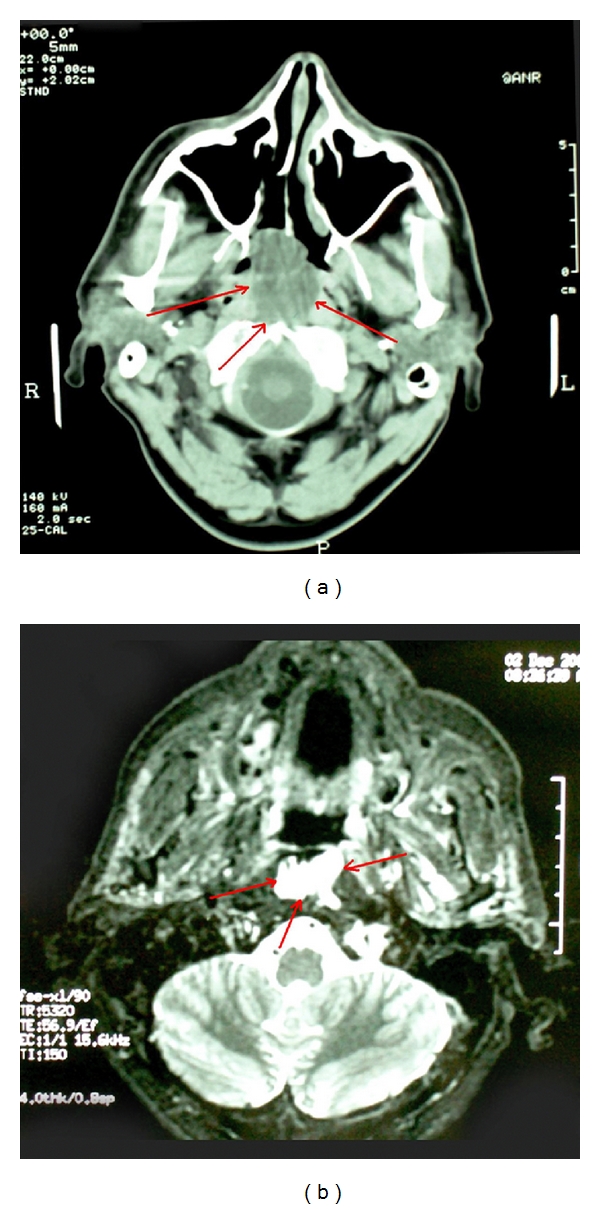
CT (a) and MRI (b) imaging of the tumor (red arrows).
